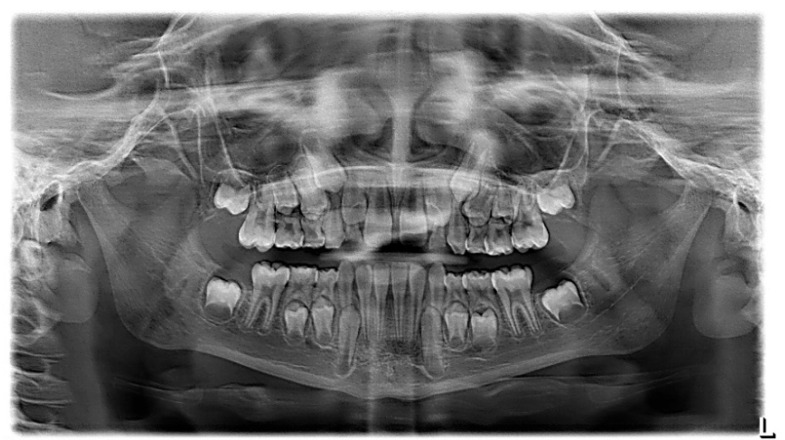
Orthopantomography X-ray before treatment (clinical case #1).
